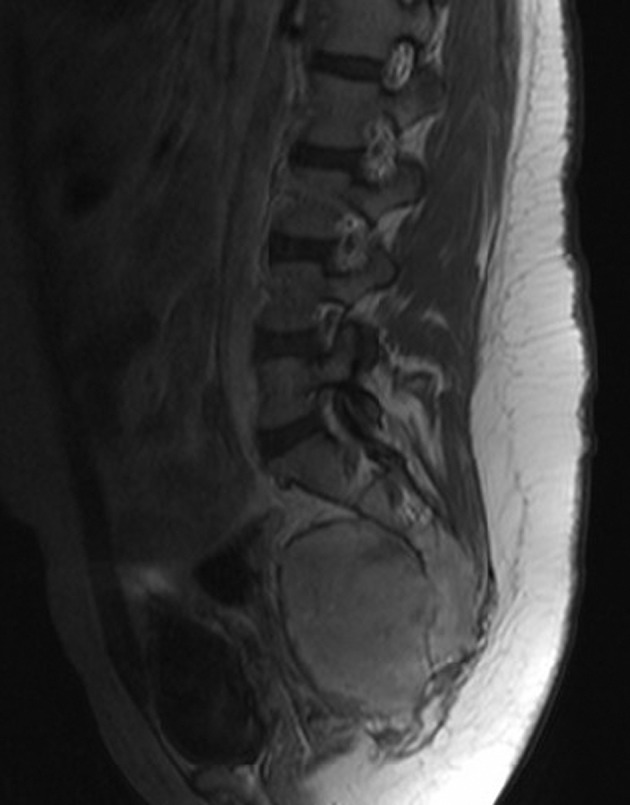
Contrast-enhanced sagittal MR T1-weighted imaging shows marked enhancement of the mass.
